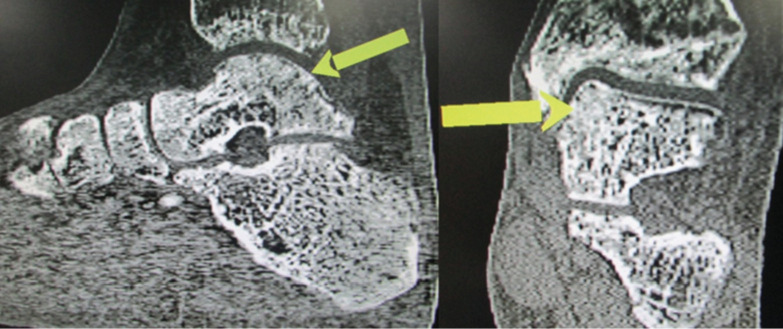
CT scan images showing healing of the subchondral cyst (Arrows- healed areas of subchondral cyst).
